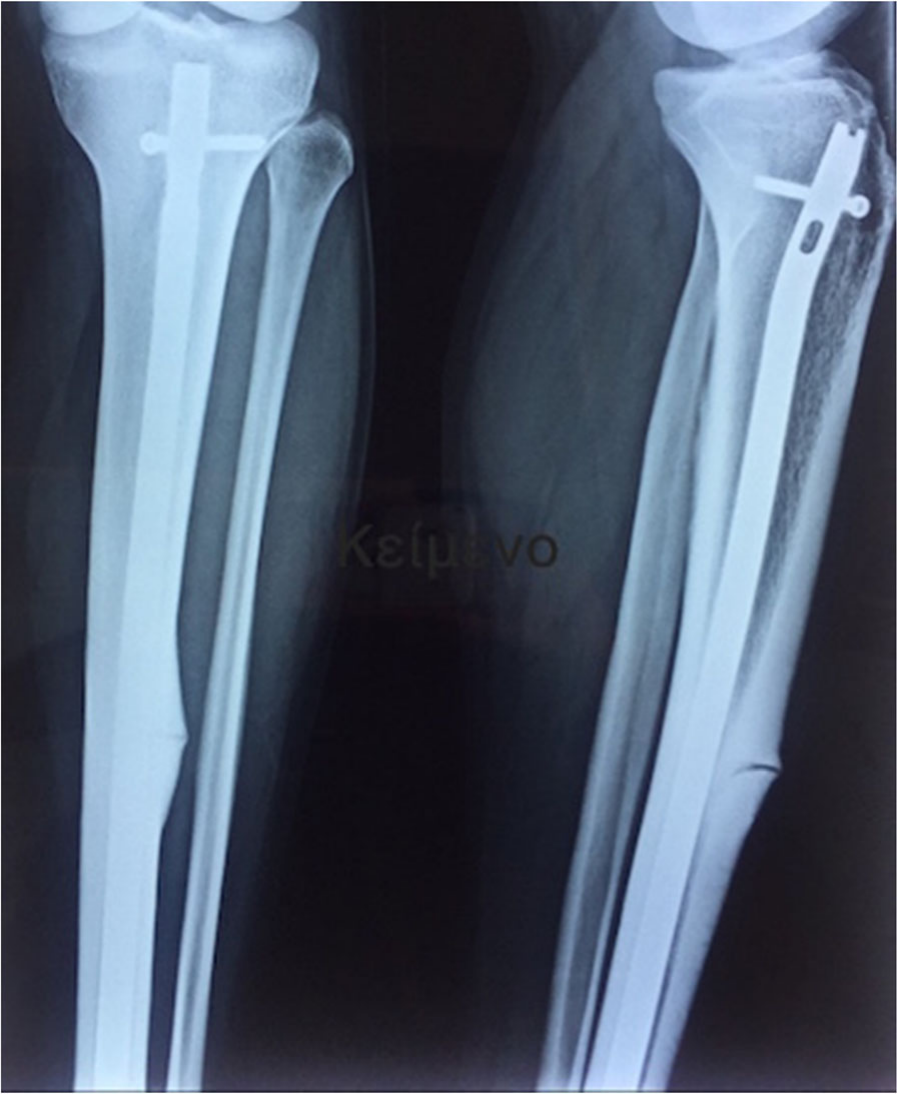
Anteroposterior and lateral X-ray of the tibia, 18 months after intramedullary nailing. The presence of a nonunion is obvious
